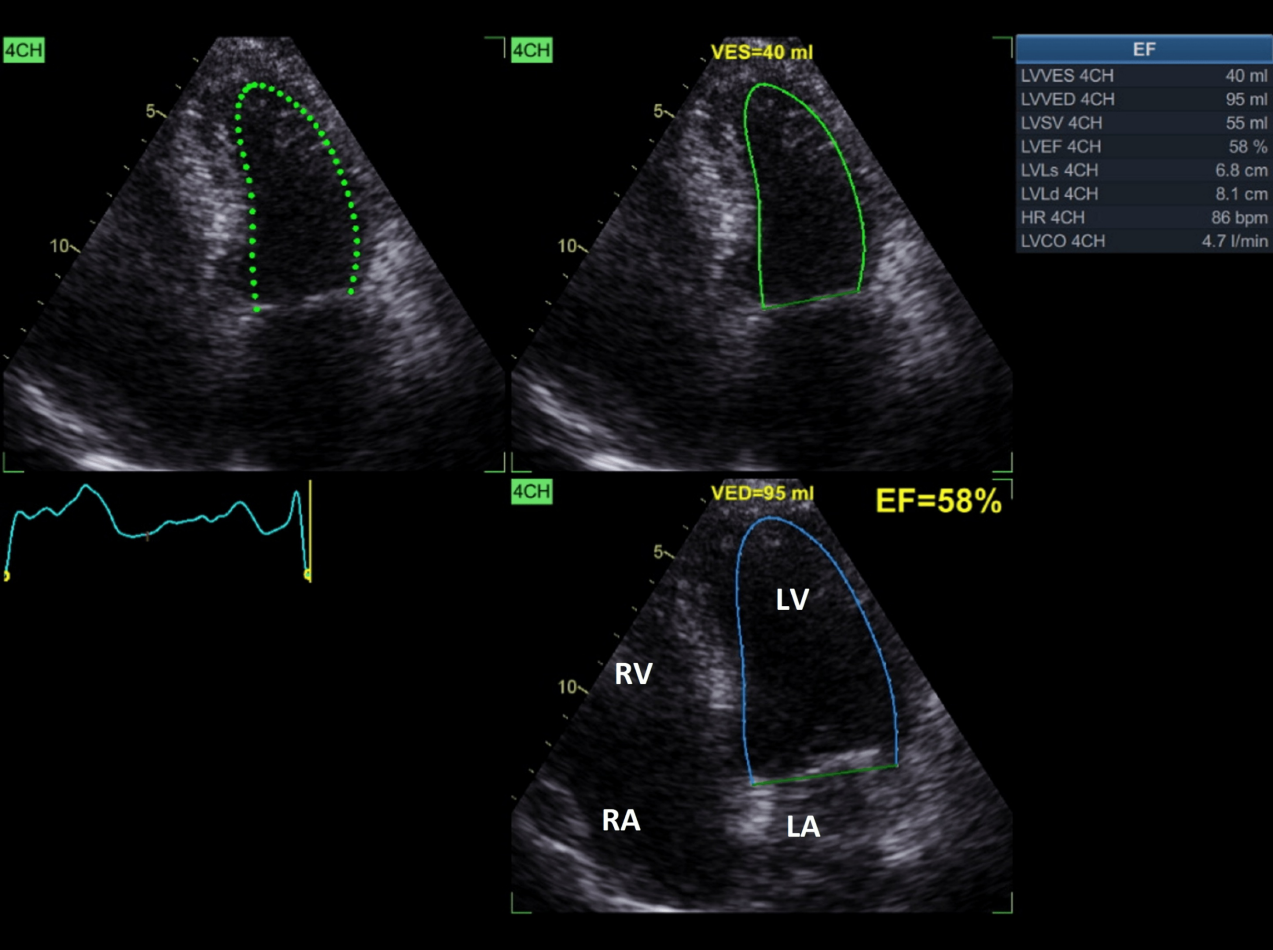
Apical four-chamber view showing automatic left ventricular ejection fraction at 58%. 4CH = four chambers; LA = left atrium; LV = left ventricle; RA = right atrium; RV = right ventricle; VED = volume end diastole; VES = volume end systole; EF = ejection fraction
